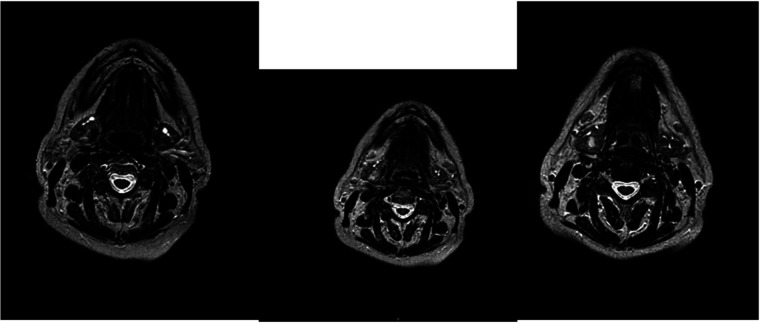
MRI with the contrast medium which highlighted the presence of an ovoid expansive process of ⁓15 mm in diameter with undefined margins compared to the surrounding glandular parenchyma. The lesion was characterized as an area with a non-homogeneous signal for T2 iso-hyperintensity and T1 iso-hypointensity with equally heterogeneous enhancement after administration of the contrast medium.
